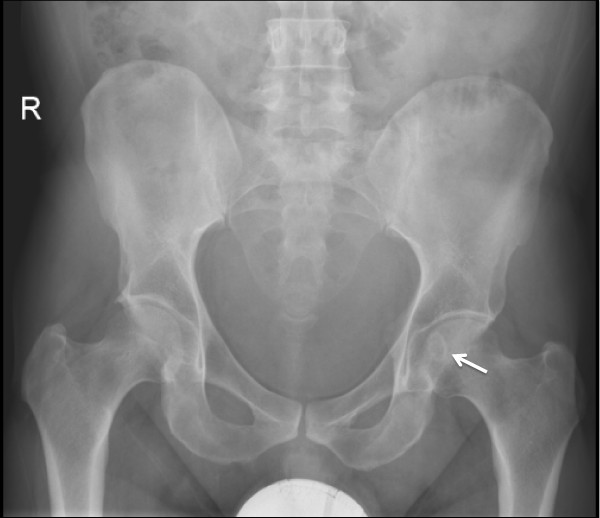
Plain radiograph in anteroposterior view showing a well defined lytic lesion with a thin sclerotic rim located in the subarticular portion of the left femoral head (white arrow).
